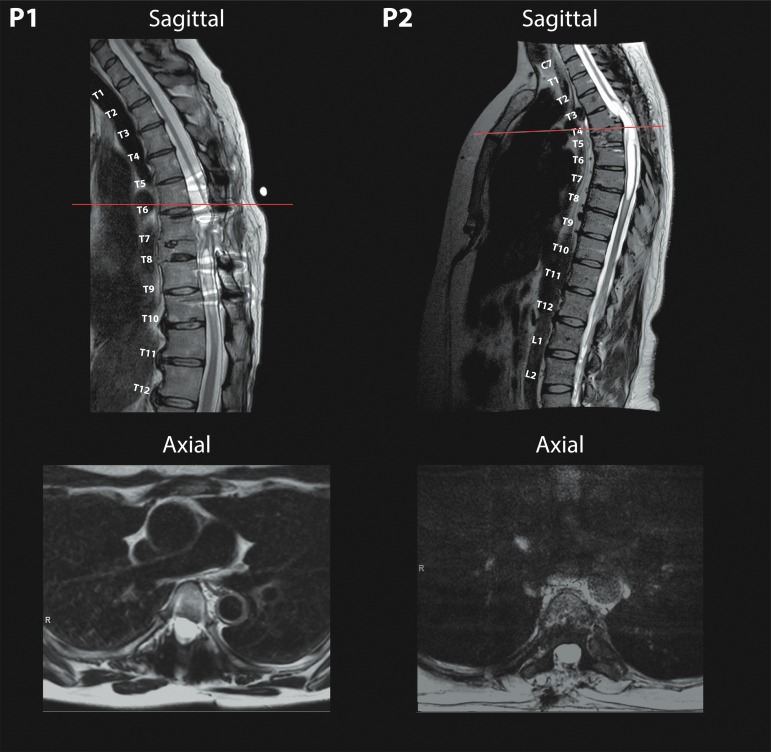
Severe spinal cord injury with associated myelomalacia in both Participant 1 (left) and Participant 2 (right) from T8 and T4/5 injuries, respectively, in midsagittal planes of T2-weighted pre-operative magnetic resonance imaging. Axial slices (bottom) demonstrate minimal remaining spinal cord tissue with corresponding scout lines present on sagittal planes.
